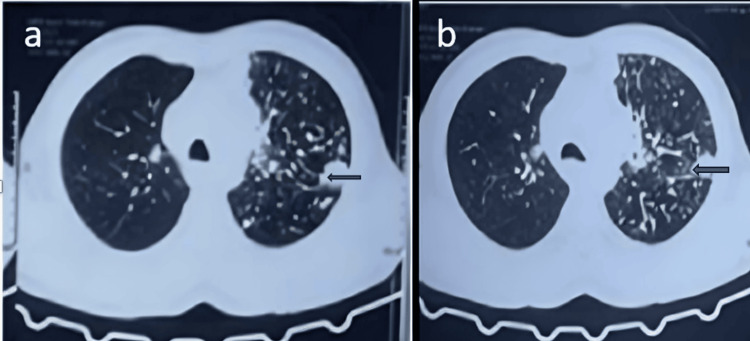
(a and b) High-resolution computed tomography (HRCT) thorax showing multiple nodular opacities in bilateral upper and lower lobes
